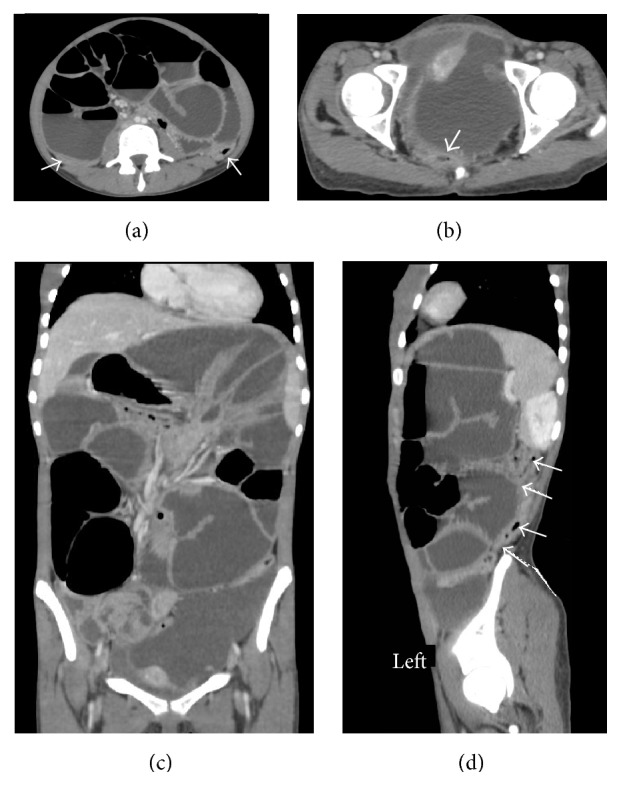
Contrast enhanced abdominopelvic CT-scan (portal phase). Axial (a, b), frontal (c), and left parasagittal (d) images demonstrated distended small bowel loops with air fluid level and mass effect on the large intestine (arrow) which is completely flat.
